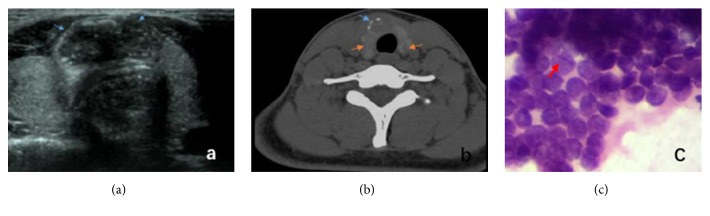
(a)* B Ultrasound*. Blue arrow for the low echo tubercle in the thyroid isthmus. (b)* CT Scan*. Yellow arrow for thyroid multiple lower density nodule; blue arrow for calcification. (c)* FNA*. Red arrow for cell nucleus ditch.
